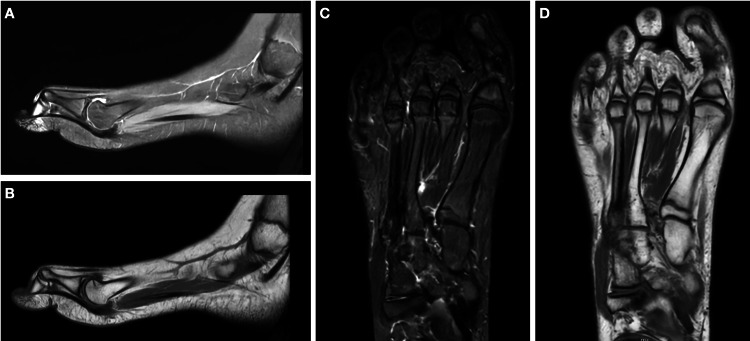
(A, B) Sagittal T2- and T1-weighted MRI scan images demonstrating a flexion of the IPJ of the right hallux with no other abnormal findings. (C, D) Axial T2- and T1-weighted MRI scan images demonstrating a flexion and valgus deviation of the IPJ of the right hallux with no other abnormal findings
